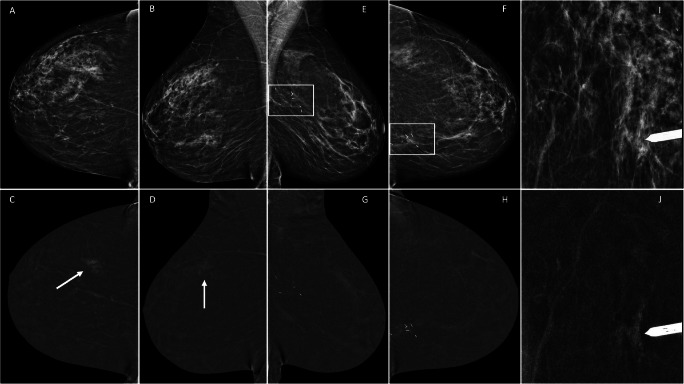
A 53-year-old patient with a history of left breast–conserving surgery was referred for complementary evaluation after questionable changes in clinical examination of the left breast. Diagnostic CEM was performed, together with ultrasound. Low-energy CEM mediolateral oblique (E) and craniocaudal (F) views of the left breast show a stable postsurgical scar (rectangle) with no enhancement at recombined images (G and H, respectively). Right breast recombined images (C and D) show an incidental 26-mm non-mass enhancing finding (arrow) not clearly seen on low-energy images (A and B, respectively), DBT or ultrasound (not shown). Right breast CEM-guided biopsy was performed (I and J). Pathology report: High-grade triple negative ductal carcinoma in situ (DCIS). The lesion was further assessed with wire needle-guided surgical conservative treatment
